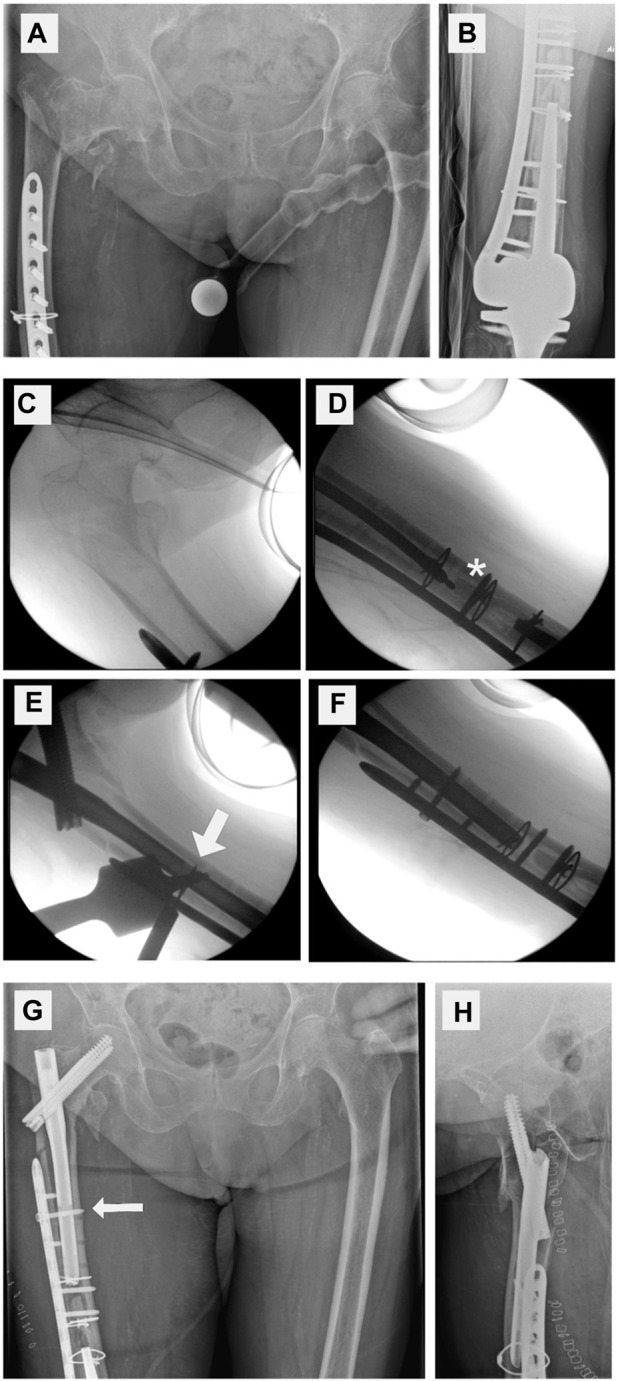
Clinical case with nail–plate docking technique. (A) Proximal femur fracture. (B) Distal locking plate. (C–F) Intraoperative fluoroscopy. Closed reduction, reaming up to bone cement (*), drilling through a plate hole and the nail (broad arrow), and placing a docking screw. Postoperative X-ray (G,H) showing the docking screw (thin arrow).
